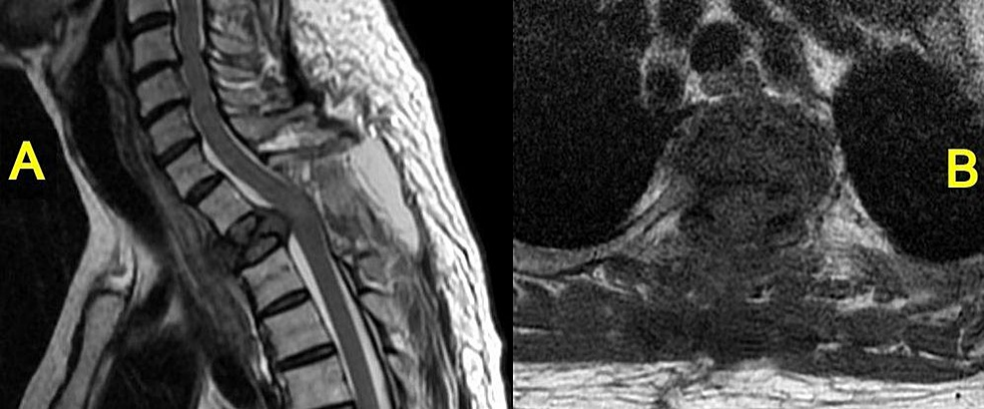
MRI on the day after start of radiotherapy (postoperative day 13). A:　T2-weighted image (sagittal)
